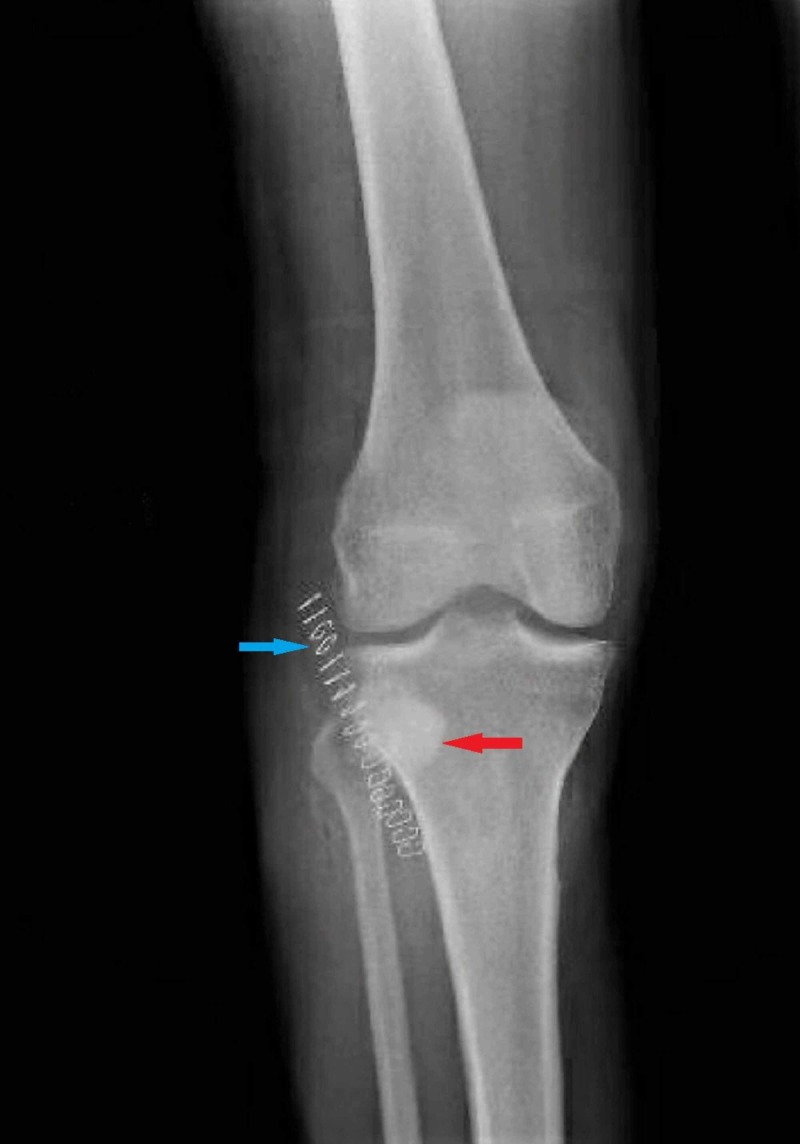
Postoperative x-ray (anteroposterior (AP) view) of the knee showing radio-opaque antibiotic cement (red arrow) in the lateral tibial condyle and staples (blue arrow)
